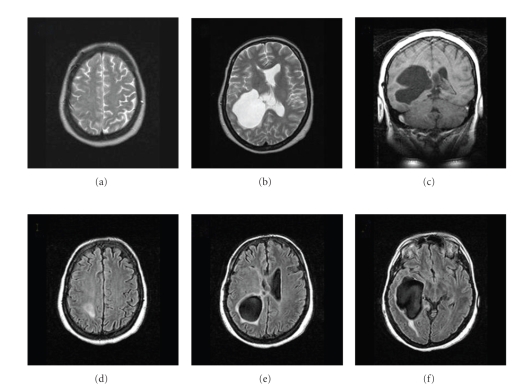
Magnetic resonance imaging (2006): (a) and (b) T2 weighted axial images; (c) T1 weighted coronal image; (d), (e), and (f) axial FLAIR (Fluid Attenuated Inversion Recovery) images. There are obstructive hydrocephalus and ventricular deformities, suggestive of intraventricular cystic lesions.
